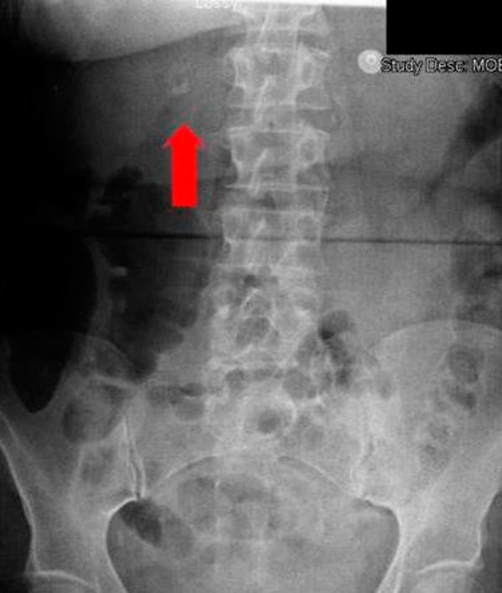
Plain erect chest radiograph, on close inspection a ring calcification can be seen in the right upper quadrant.
